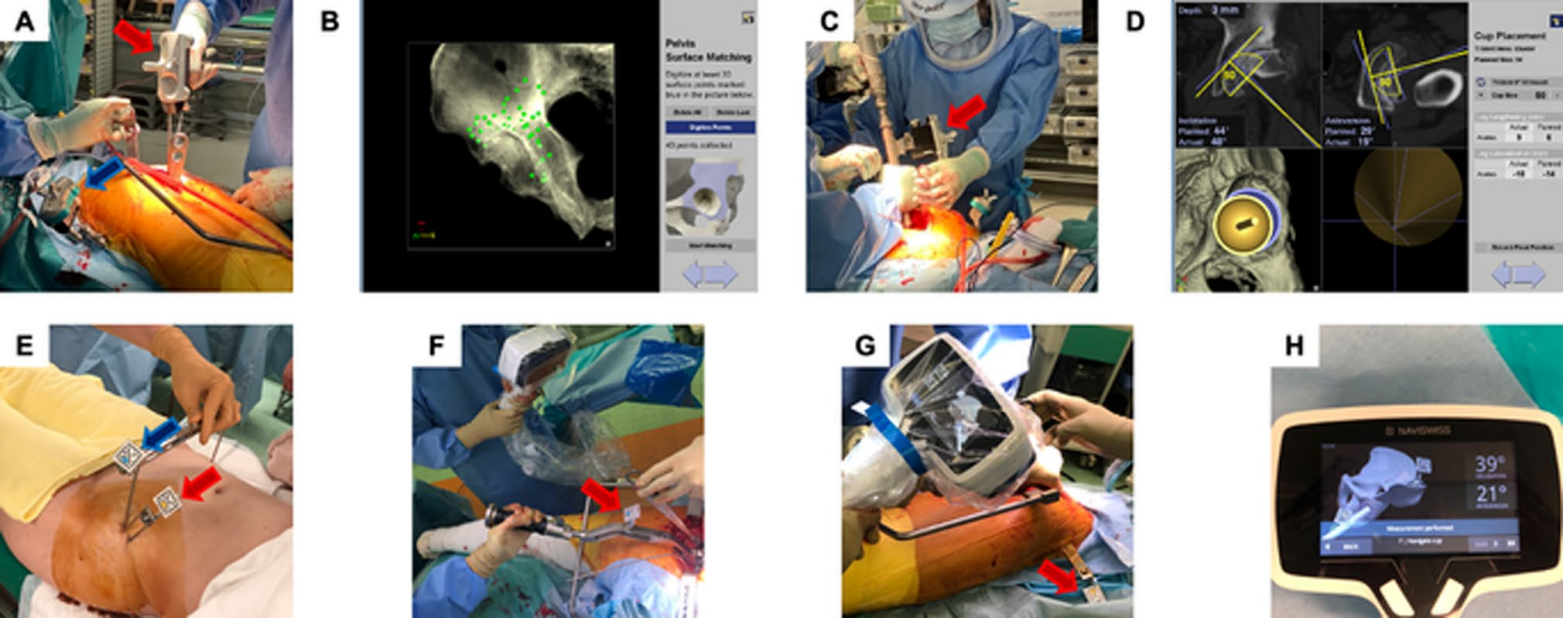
CT-based navigation system and portable navigation system. A CT-based navigation system. Intraoperative image during registration. The pelvic surface is palpated with a pointer (Indicated by the red arrow). The tracker is attached to the iliac crest (Indicated by the blue arrow). B Navigation screen during surface registration. C Navigation attached to the reamer (Indicated by the red arrow). D Navigation screen during cup placement. The angle and position of cup placement can be checked. E Portable navigation system. Registration is performed prior to surgery. The P-tag is attached to the pelvis (Indicated by the red arrow); both superior anterior iliac spines are palpated using a pelvic caliper with the M-tag (Indicated by the blue arrow). F The M-tag is attached to the cup holder (Indicated by the red arrow). G The P-tag is attached to the pelvis (Indicated by the red arrow). H Only the angle of cup placement can be checked intraoperatively
